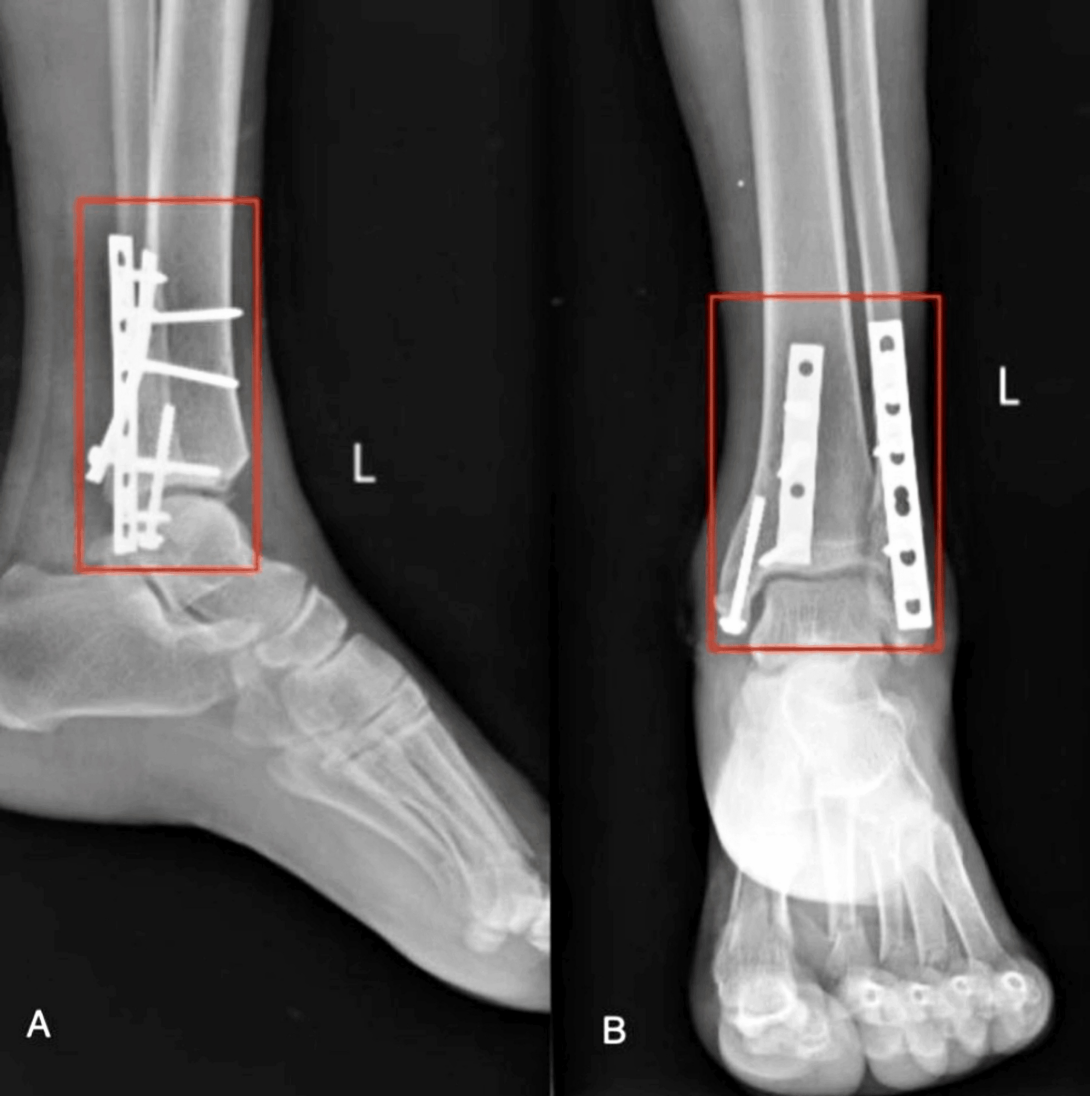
Postoperative X-ray of the left ankle joint AP view AP - antero-posterior A) Semi-tubular plate 6 hole, cortical screw; B) Semi-tubular plate 8 hole, cortical screw
